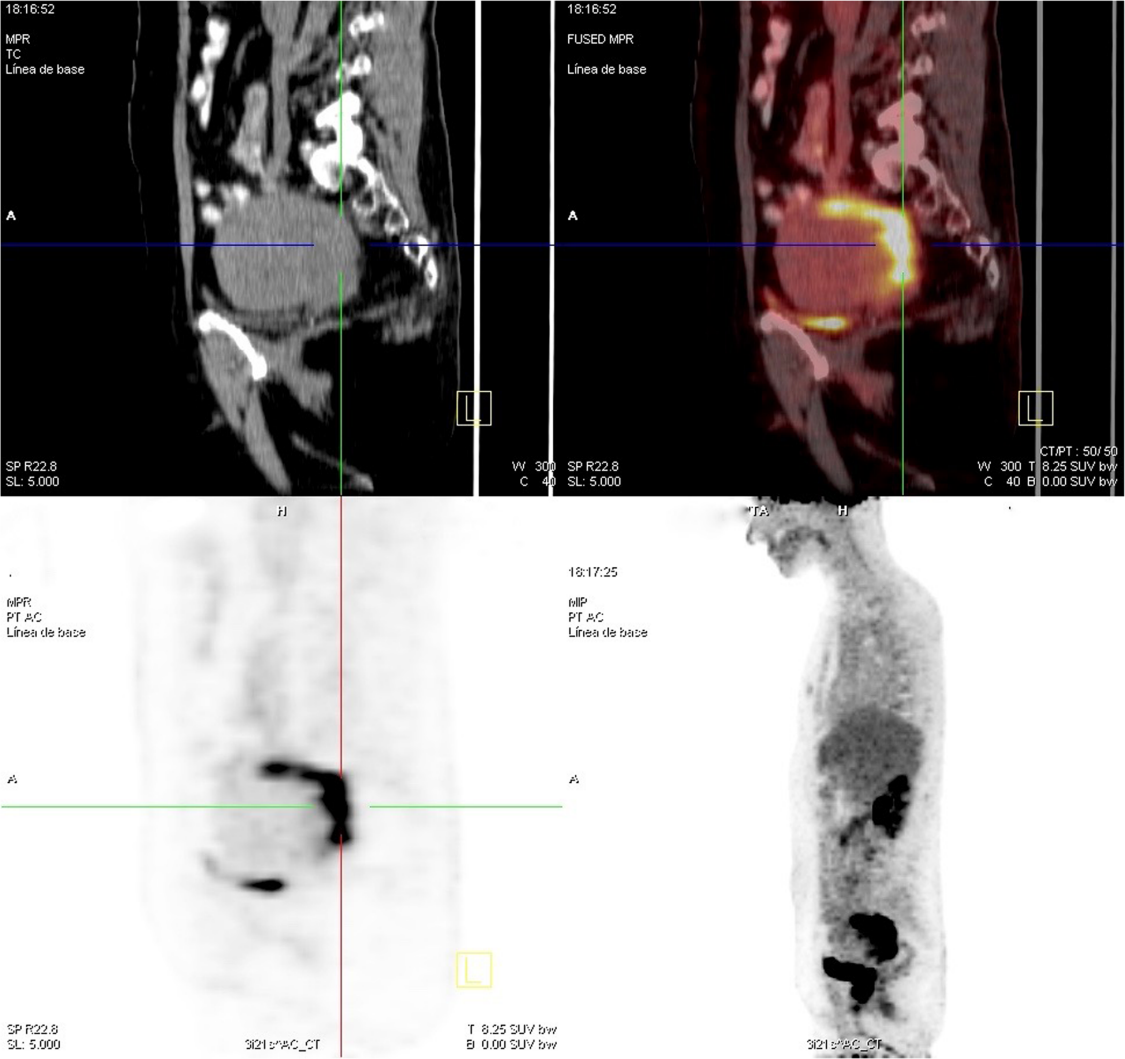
FDG-PET/CT demonstrates increased FDG uptake by the tumoral lesions on uterine corpus and vagina
